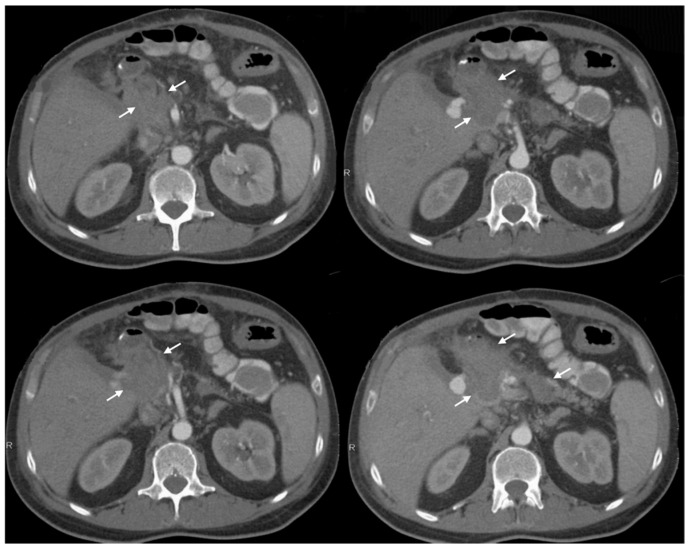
CT Angiography of Right Hepatic Artery Pseudoaneurysm in HIAA. Contrast-enhanced CT imaging of a patient with hepaticojejunostomy insufficiency-associated arterial hemorrhage demonstrating a large hematoma (white arrows) secondary to pseudoaneurysmal bleeding of the right hepatic artery. Due to severe stenosis at the origin of the celiac trunk, endovascular intervention was not feasible and immediate surgical management was required.
